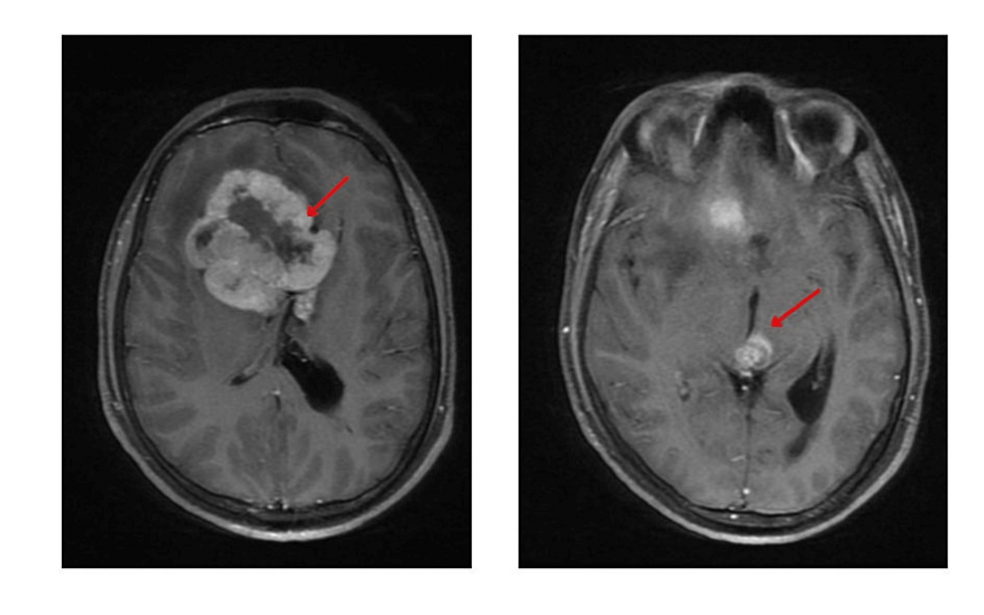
Pretreatment MRI showing the lesions
